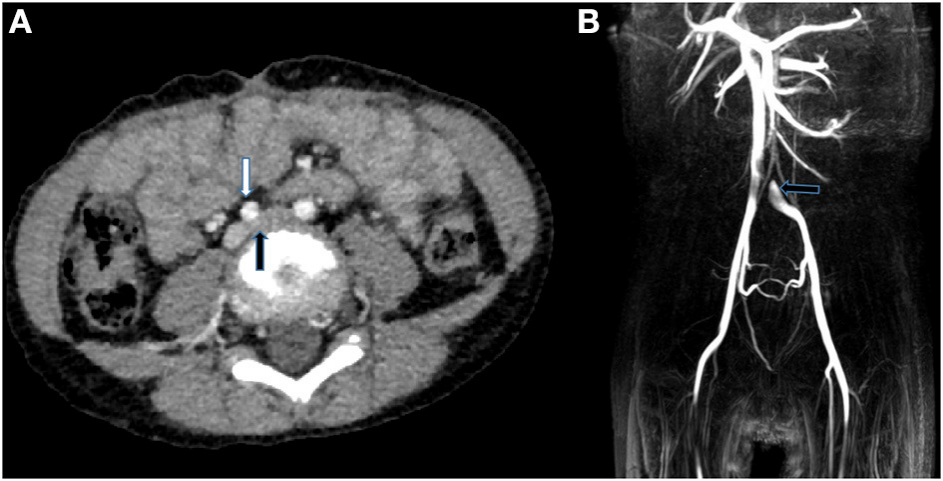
(A) CTA in the transverse plane showed the left common iliac vein (black arrows) was compressed between the right common iliac artery (white arrow) and the lumbar vertebral body. (B) MRI images indicated that there was filling defect where the left common iliac vein merged into the right common iliac vein.
